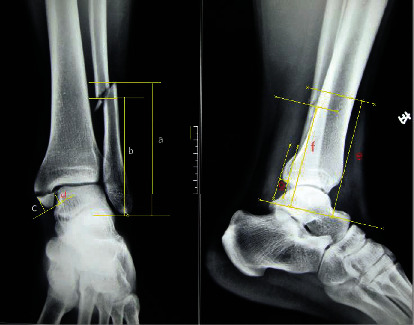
The fracture line of the medial and lateral malleoli obtained from the typical PAB stage III ankle plain radiographs using Digitizer 4.2.6.0. (i) Anteroposterior ankle view: (a) the lateral height of the lateral malleolus; (b) the medial height of the lateral malleolus; (c) the medial height of the medial malleolus; (d) the lateral height of the medial malleolus. (ii) Lateral ankle view: (e, f) the measurement of the lateral malleoli, which could indicate the fracture line trend.
